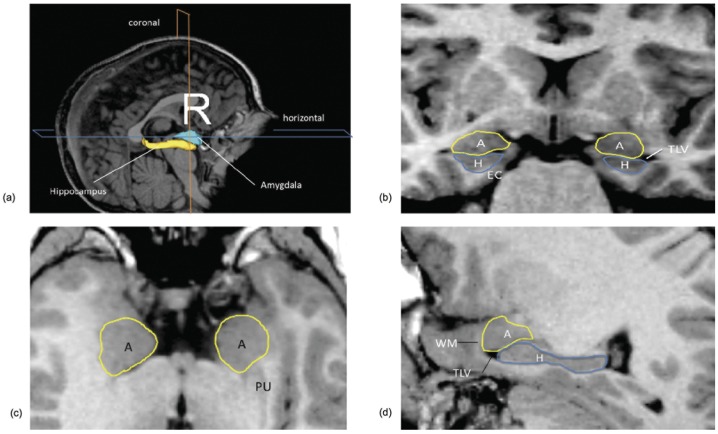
Orthogonal views for segmenting the amygdala and hippocampus on MRI sections. A three-dimensional reconstruction of images (a) in which lines indicate the position of the horizontal plane (b), sagittal plane (c), and coronal plane (d) is shown. A, Amygdala; EC, entorhinal cortex; H, hippocampus; PU, putamen; TLV, temporal horn of the lateral ventricle; WM, subamygdaloid white matter.
